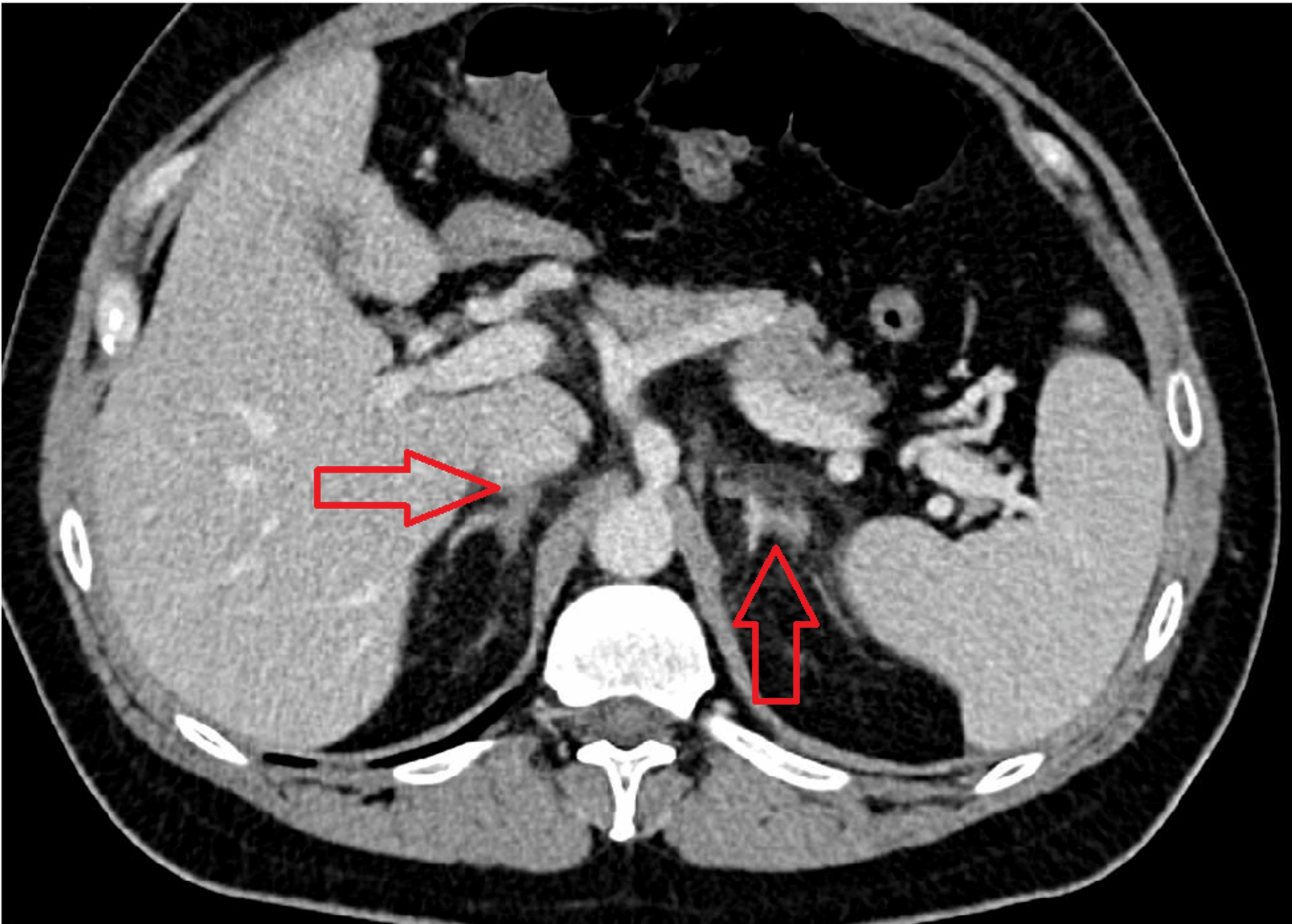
Axial contrast-enhanced CT image of the upper abdomen showing bilateral adrenal gland thickening with periadrenal fat stranding (arrows), consistent with nonhemorrhagic adrenalitis
